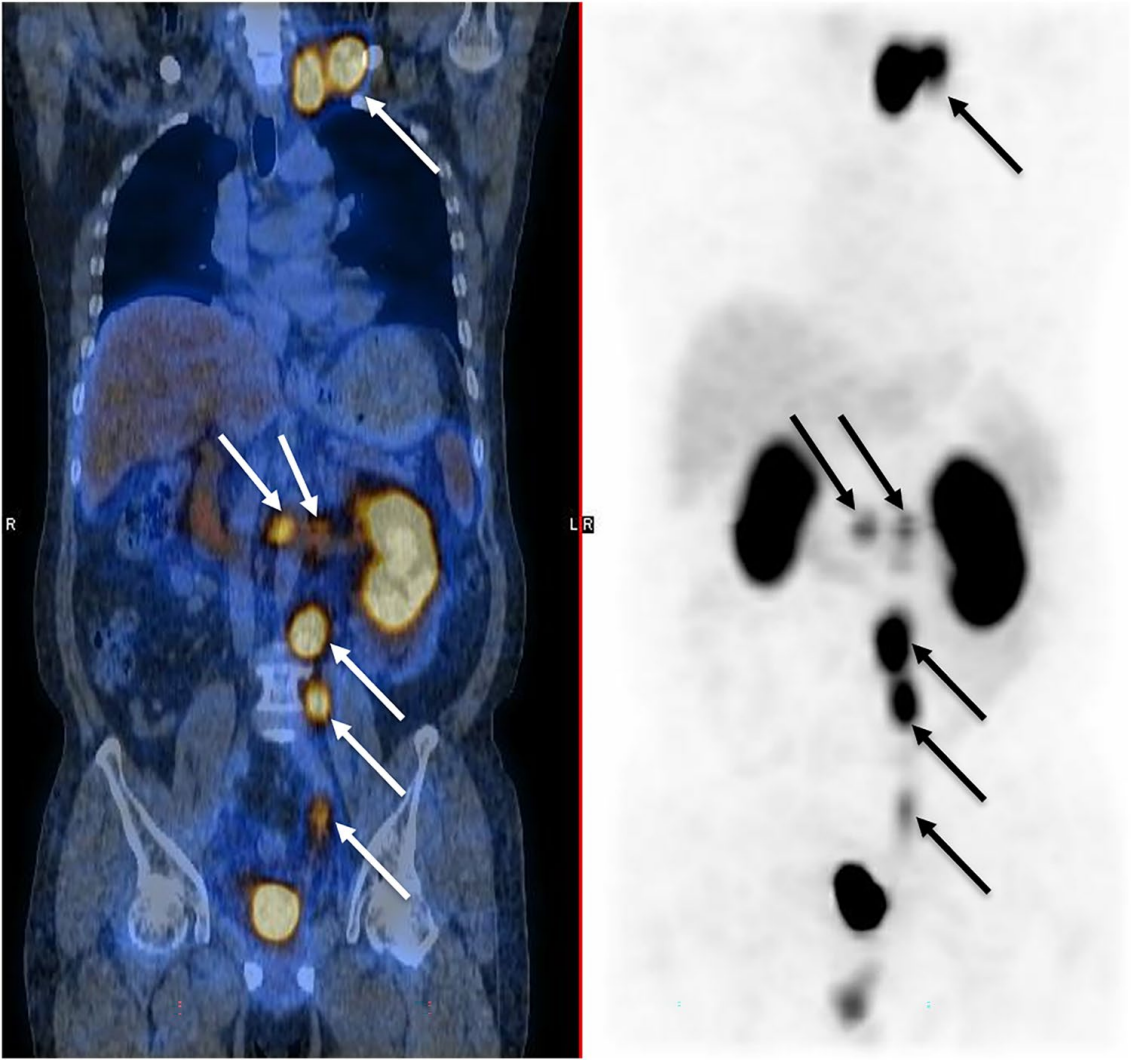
Maximum intensity projection (right) and fused (left) ^99m^Tc-HYNIC-PSMA SPECT/CT images of a 59-year-old patient, who previously received a radical prostatectomy, 2 h after administration of 740 MBq dose. Multiple lymph node metastases were detected (arrowed) and the left supraclavicular lymph node has a maximum tumor-to-background ratio of 9.2.
